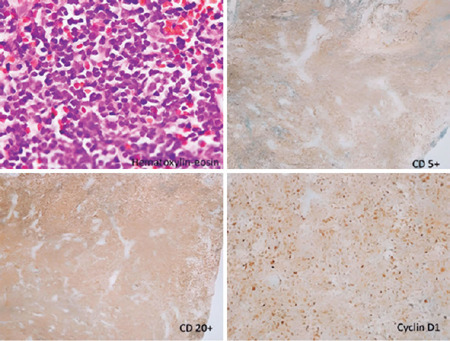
Orbital CT imaging shows a well-defined hypodense soft tissue mass in the right
